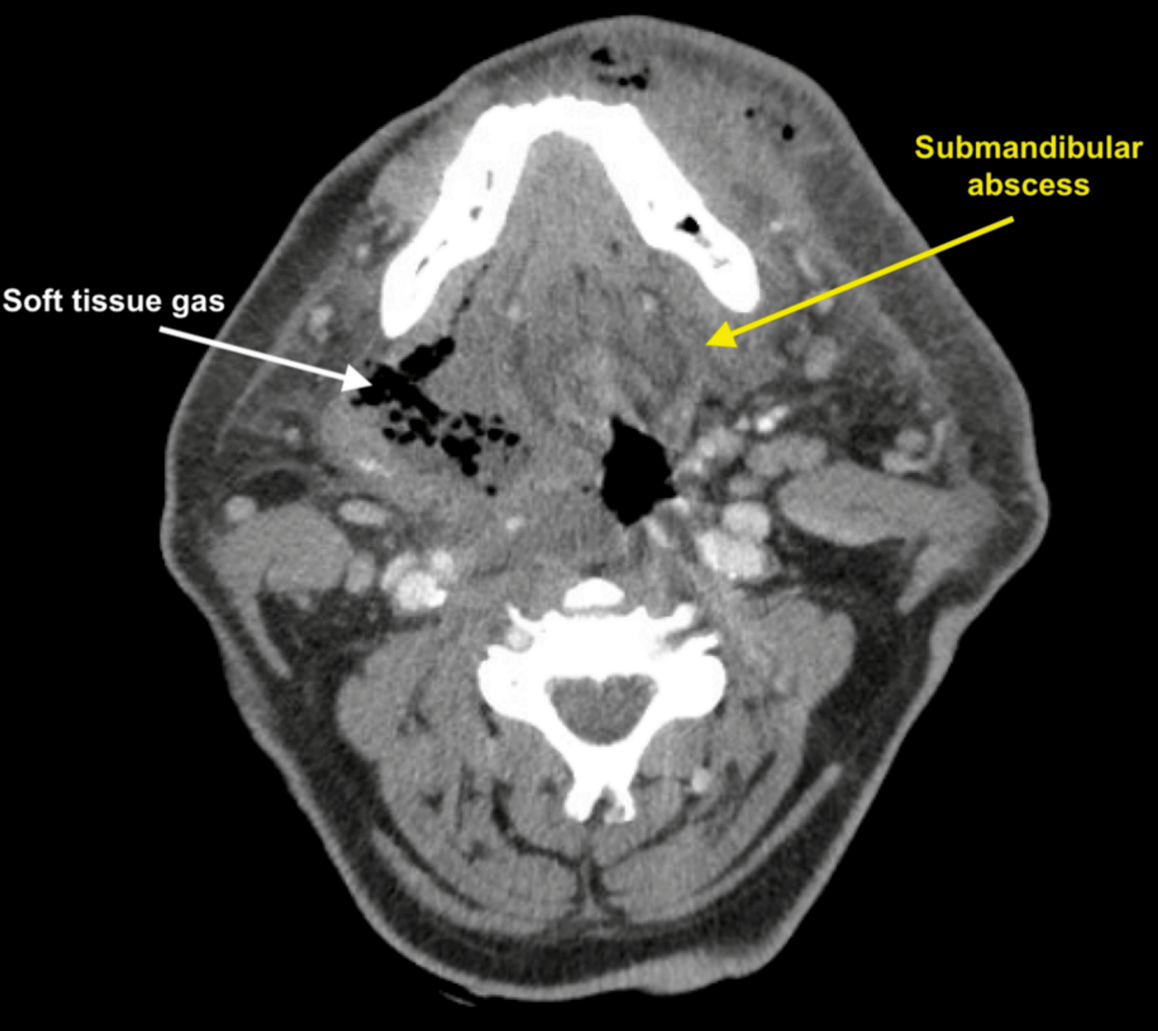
Pre-operative CT soft tissue neck An ill-defined collection of fluid and air, and associated skin thickening at the left anteromandible, along with a submandibular abscess (yellow arrow). There is extensive fat stranding seen in this region. Additionally, soft tissue subcutaneous air is seen within the right floor of the mouth and along the musculature of the floor of the mouth (white arrow).
